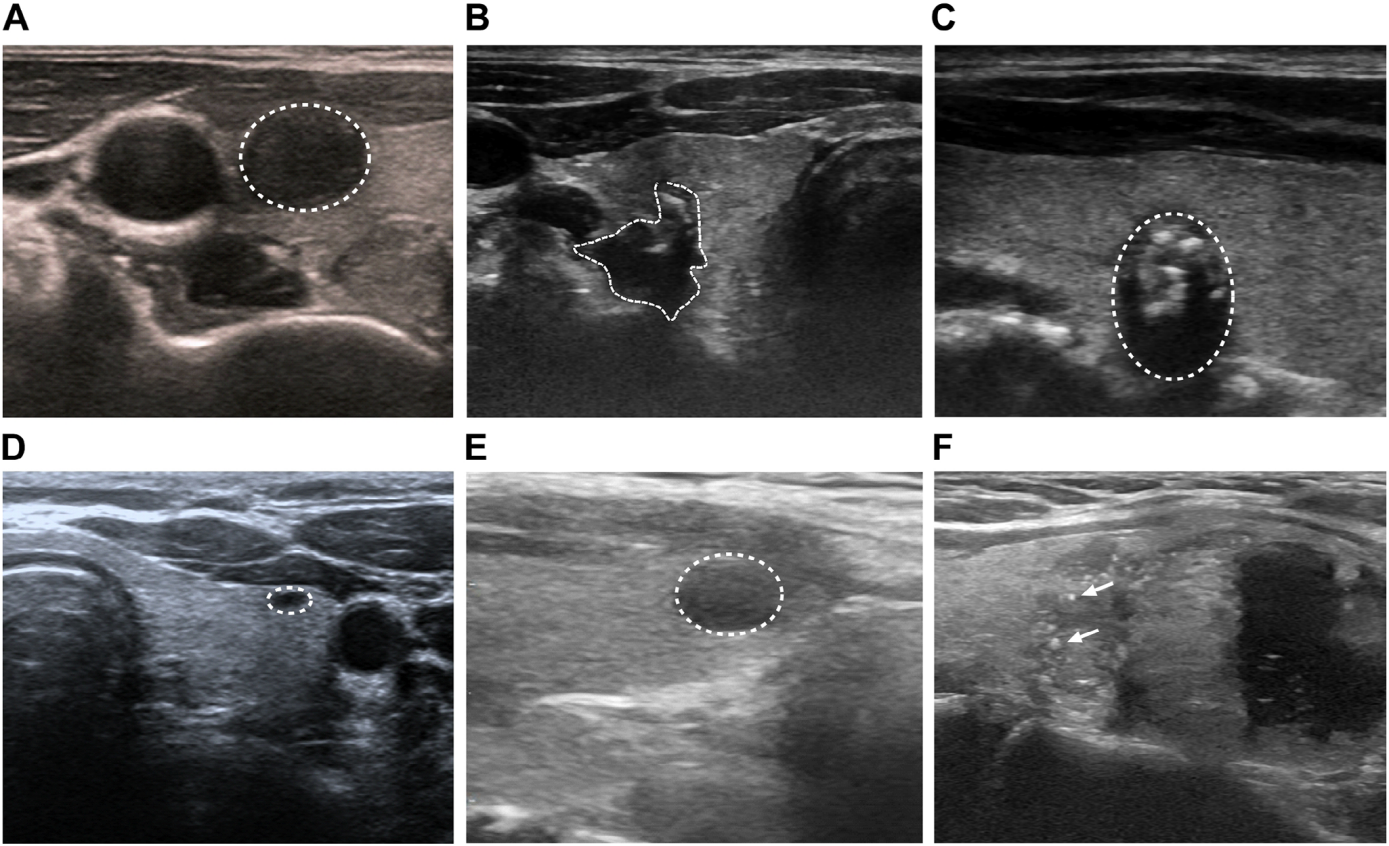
The features of ultrasound imaging. (A) Regular margin; (B) irregular margin; (C) taller‐than‐wide shape: the anteroposterior diameter is greater than its transverse diameter; (E) hypoechogenicity; (F) medium echogenicity; (G) punctate hyperechoic foci.
